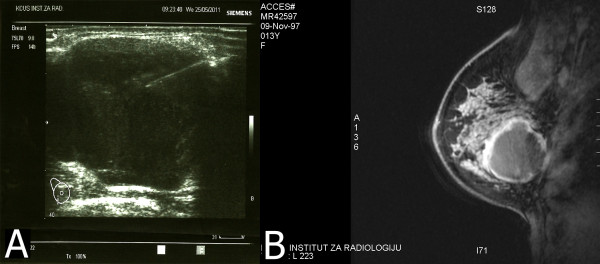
Ultrasound examination (A) and MRI (B) of the breast (Case #1) revealed a circumscribed, hypoechoic mass, measuring 40 mm.
